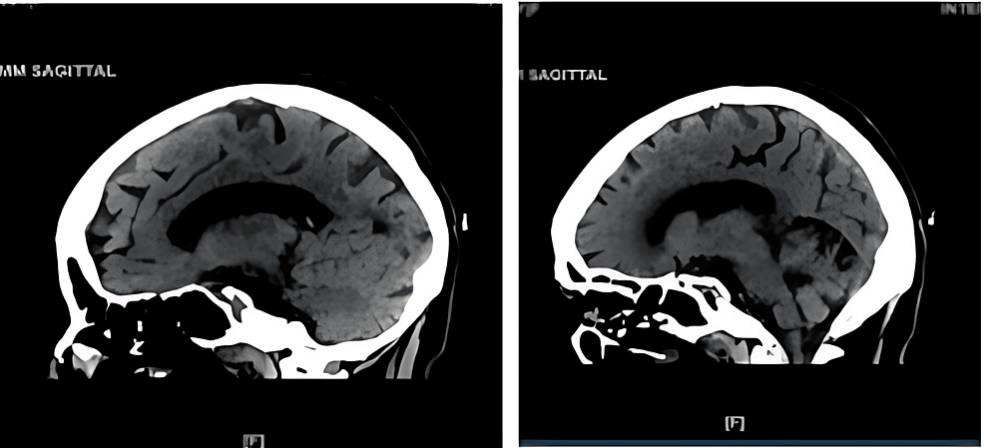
Sagittal CT without contrast scan of head done in 2022.
